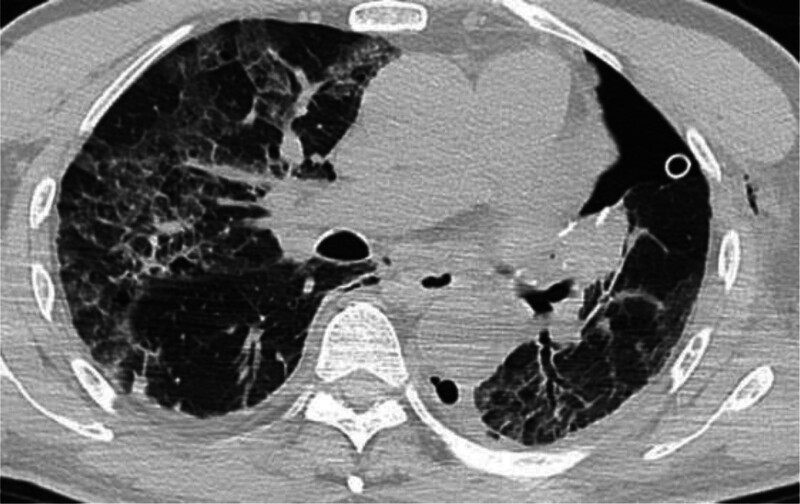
The results of a chest CT in our hospital on May 27, 2021.
